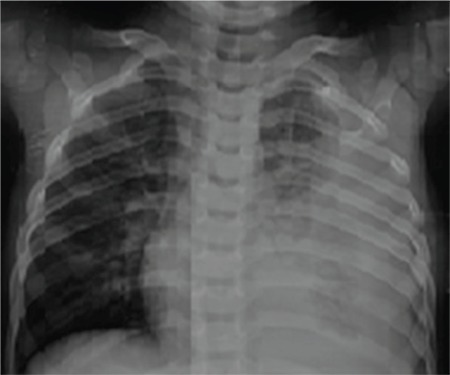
Pleural effusion and consolidation on chest X-ray of the patient.
